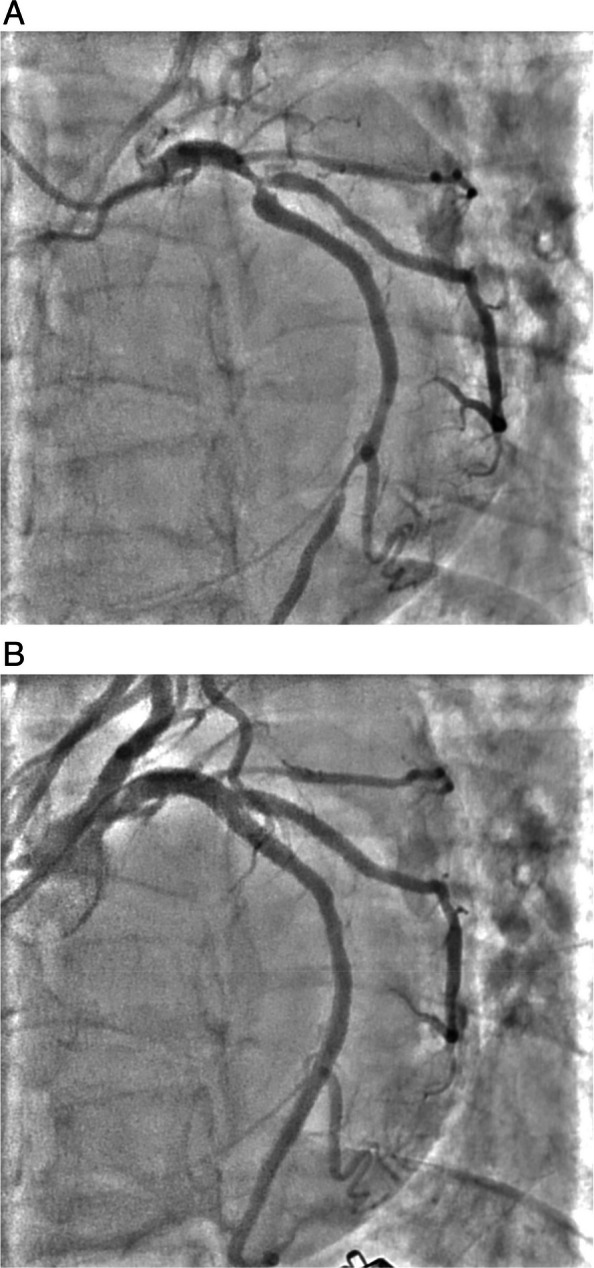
A 72-year-old male patient was admitted to the emergency department with severe angina and was diagnosed with non–ST-segment elevation myocardial infarction. A, B Transthoracic echocardiography demonstrated a left ventricular ejection fraction of 30%, a left ventricular end-diastolic diameter of 59 mm, and severe functional mitral regurgitation (Carpentier type IIIb). The patient underwent percutaneous coronary intervention with implantation of two DynamX (Elixir Medical Corp) drug-eluting stents (3.5 × 28 and 3.5 × 23 mm) using the culotte technique
